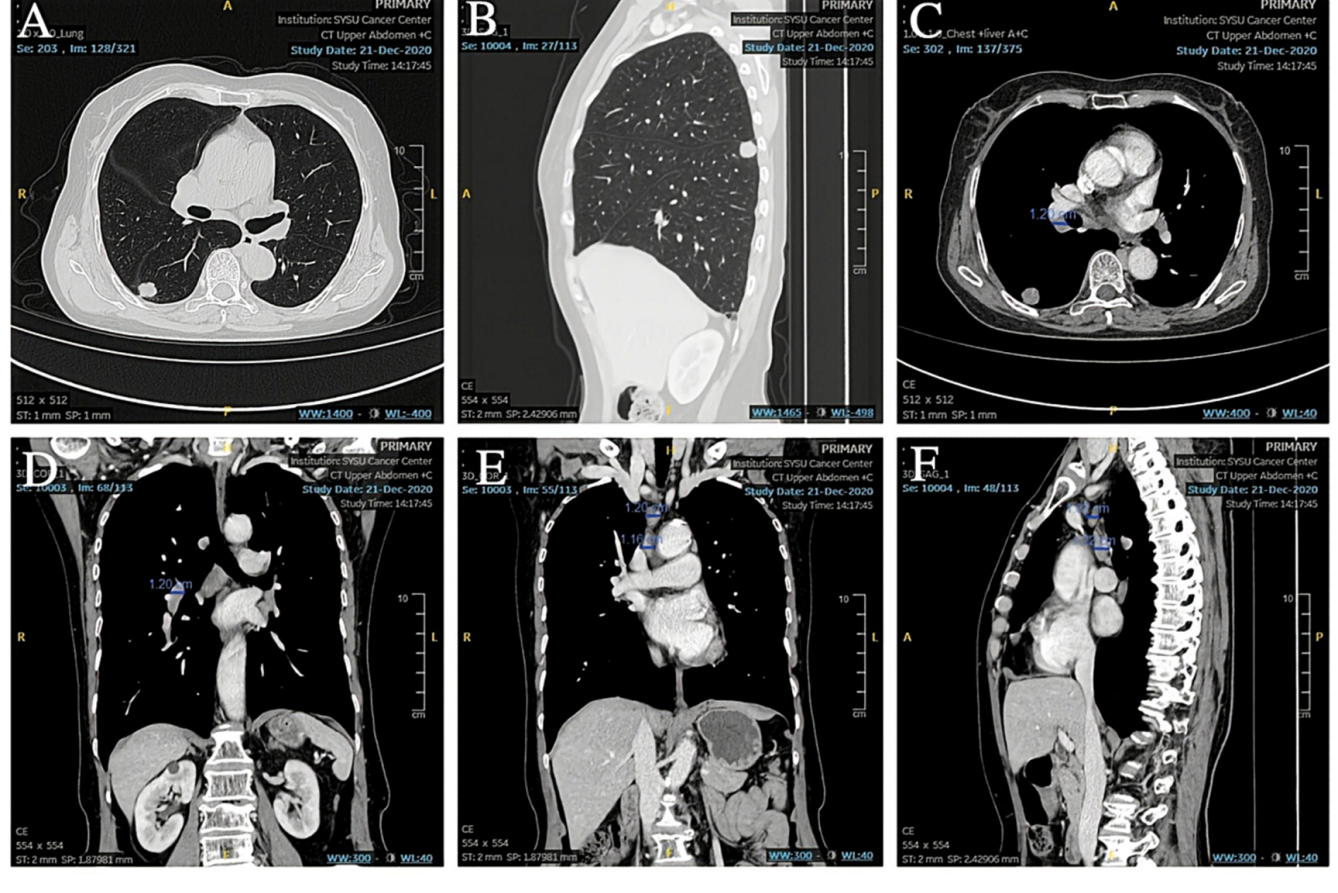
(A,B) Preoperative CT showed a space-occupying lesion in the lower lobe of the right lung. (C,D) Preoperative CT showed hilar lymph nodes with a short diameter less than 1 cm. (E,F) Preoperative CT showed other lymph nodes in the mediastinal region.
